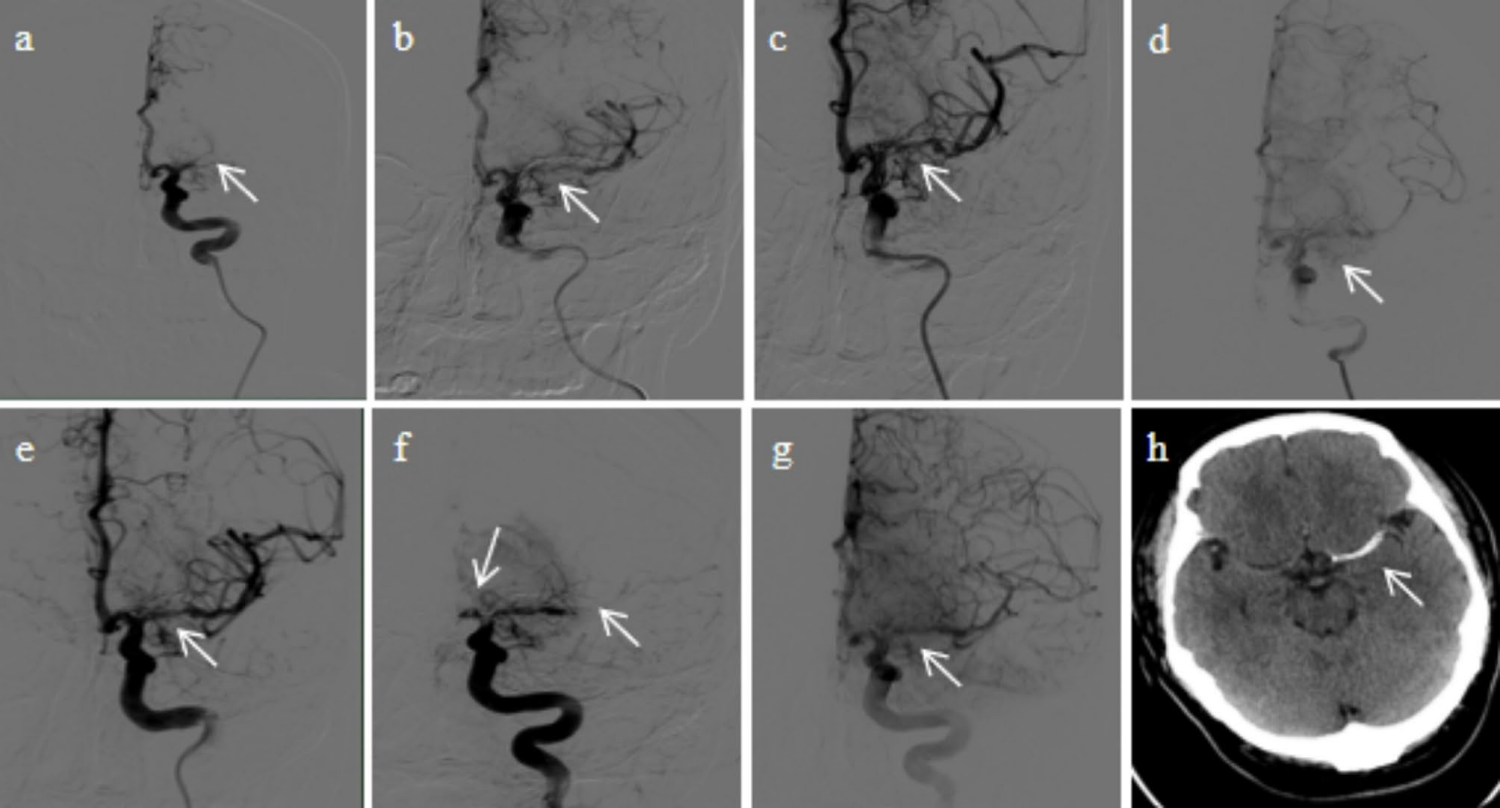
Intraoperative Images. a Left M1 occlusion. b A solitaire stent (4 mm×20 mm) was placed in the occluded segment and the left MCA M1 was recanalized. c Post-mechanical thrombectomy angiogram revealed the left MCA is unobstructed. d Repeated carotid angiography revealed the left MCA is hemodynamically unstable with mTICI grade 2a. e Recanalization of left MCA M1 segment after stent implantation. f The angiography showed mass thrombosis in the stent, ACA and left MCA occluded. g Angiography showed recanalization of the ACA and left MCA. h Post-procedure CT brain showed no hemorrhage and a stent in the M1 segment of the left MCA
